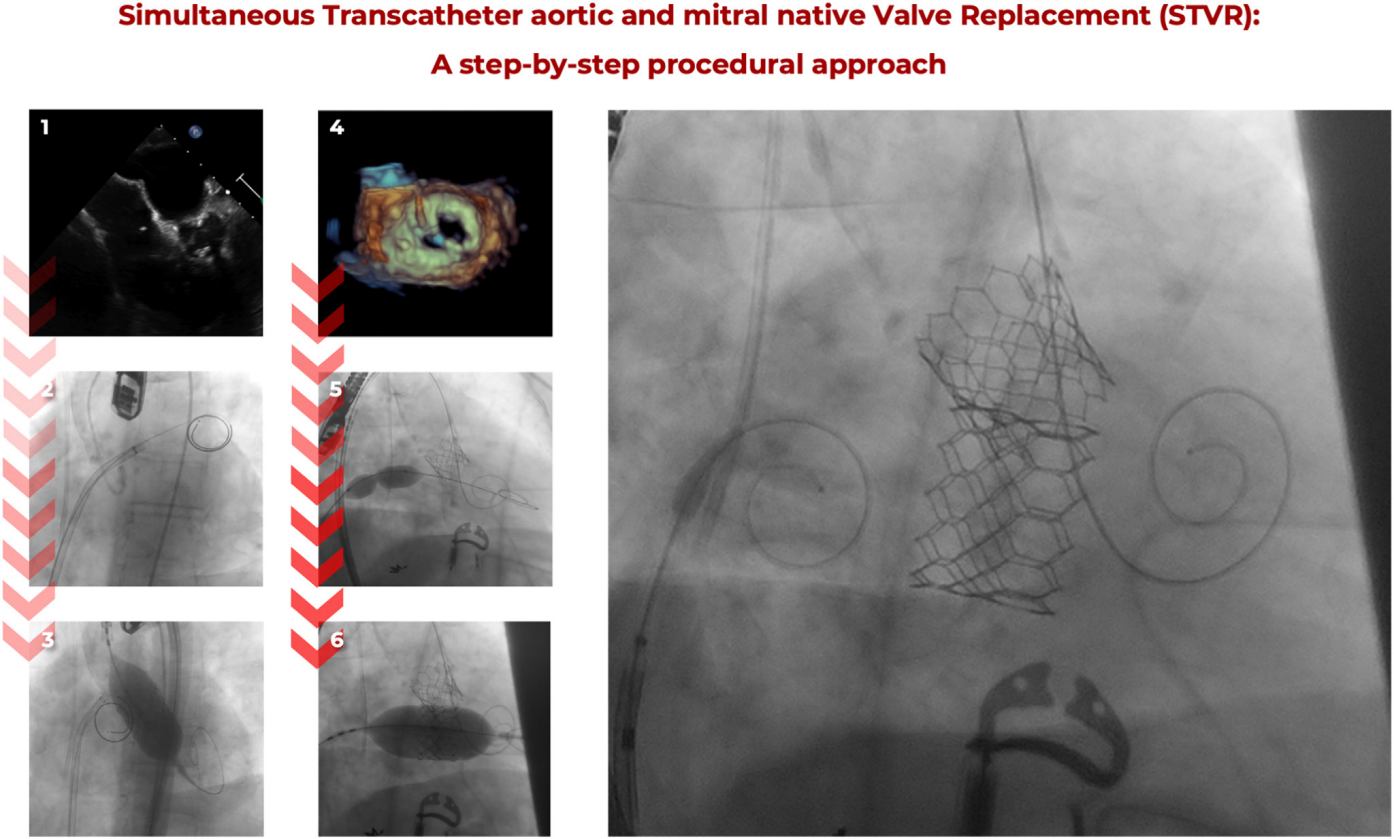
Simultaneous Transcatheter aortic and mitral native valve replacement (STVR). After setting vascular accesses (main TAVR-main access into the left femoral artery, main TMVR-main access into the right femoral vein and secondary TAVR access into the right femoral artery) and after giving half-dose heparin, the first STVR procedural step (1) is performing the TEE-guided transeptal puncture and then complete the heparin dose. Leaving the transeptal puncture system in place, the pre-TAVR aortogram is performed (2) to finally deploy the aortic THV (3) while holding the LV wire into the LV after the aortic THV deployment. Under TEE guidance, the native mitral valve is crossed (4) and access into the LV is secured with the upwardly directed curve of the LV wire. The mitral valve and then interatrial septum predilation (5) are performed. Then, the mitral THV slow deployment (6) is performed. Both valves are deployed under rapid pacing using the LV wire. Abbreviations: LV, left vetnricle; STVR, simultaneous transcatheter valve replacement; TAVR, transcatheter aortic valve replacement; TEE, transesophageal echocardiography; THV, transcatheter heart valve; TMVR, transcatheter mitral valve replacement.
